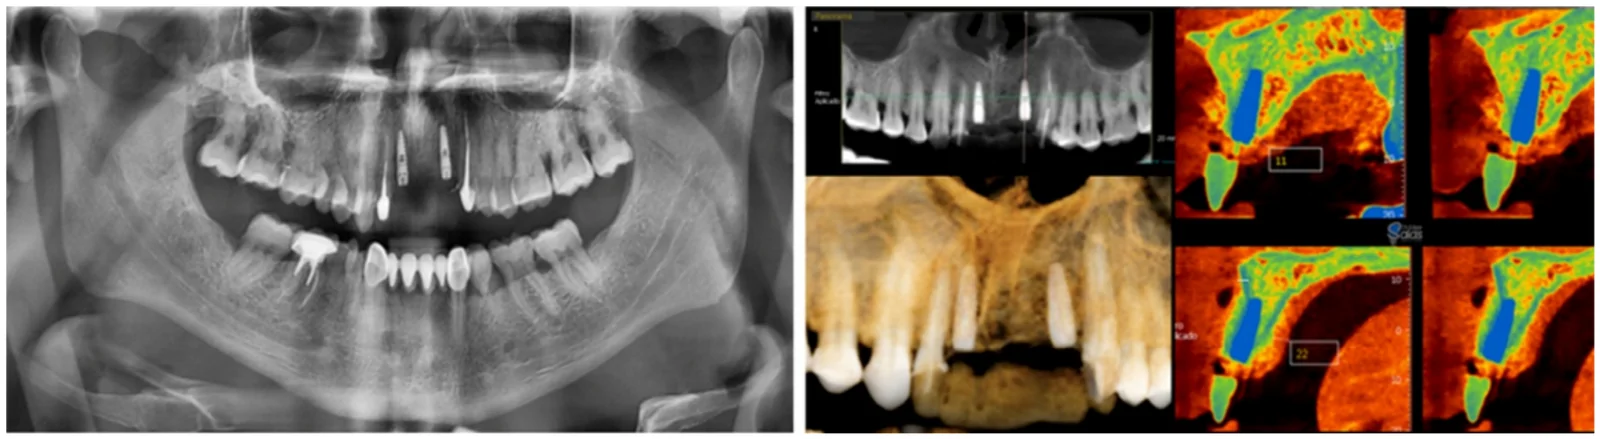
Four-month postoperative panoramic radiograph (left) and CBCT images ((middle) and (right)) demonstrating successful ridge augmentation and bone formation around the implants, colors indicate variances in density.
